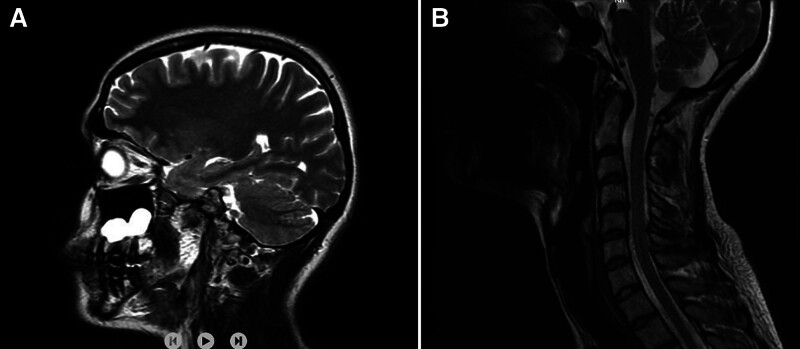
Cranial MRI of the patient (A). Sagittal section of cervical MRI of the patient showing disc bulging at C4–C5 level (B). MRI = magnetic resonance imaging.
